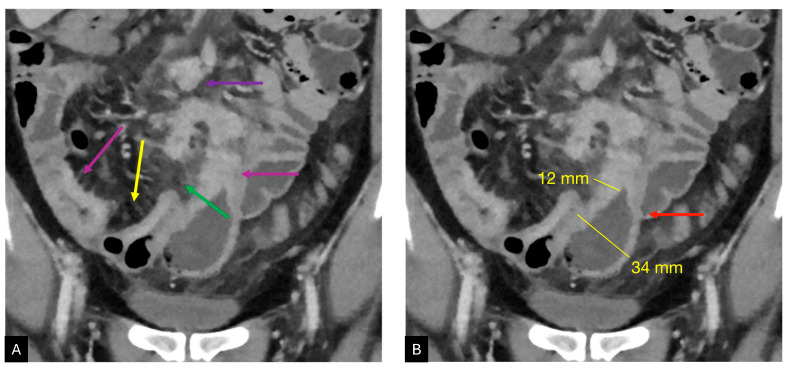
A 52-year-old male underwent CTE for bowel evaluation with a known history of Crohn’s disease. The patients reported multiple episodes of diarrhea, with increased laboratory inflammatory indexes. CT coronal multiplanar reconstruction on the portal venous phase is reported in (A,B). The most critical findings in (A) are diffuse and multiple small bowel wall thickening (red arrows) associated with comb sing (yellow arrow), peri-visceral oedema (green arrow), and enlarged nodes (purple arrow). In (B), it is possible to detect a significant thickening of a small bowel loop (red arrow), with homogeneous contrast enhancement, associated with a slight dilation of the upstream bowel portion.
